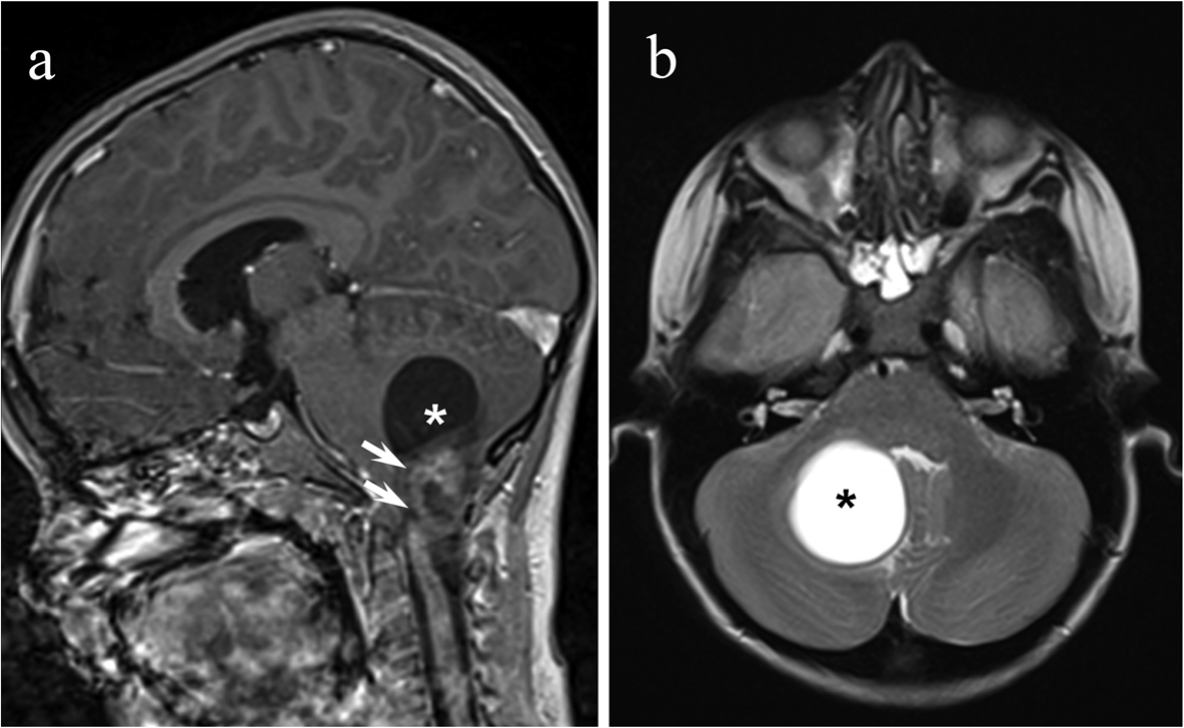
Sagittal contrast-enhanced T1-weighted (a) and axial T2-weighted (b) images showing cystic cerebellar mass (asterisk) with a large dyshomogeneous enhancing nodule (white arrows) arising from the left cerebellar hemisphere.
